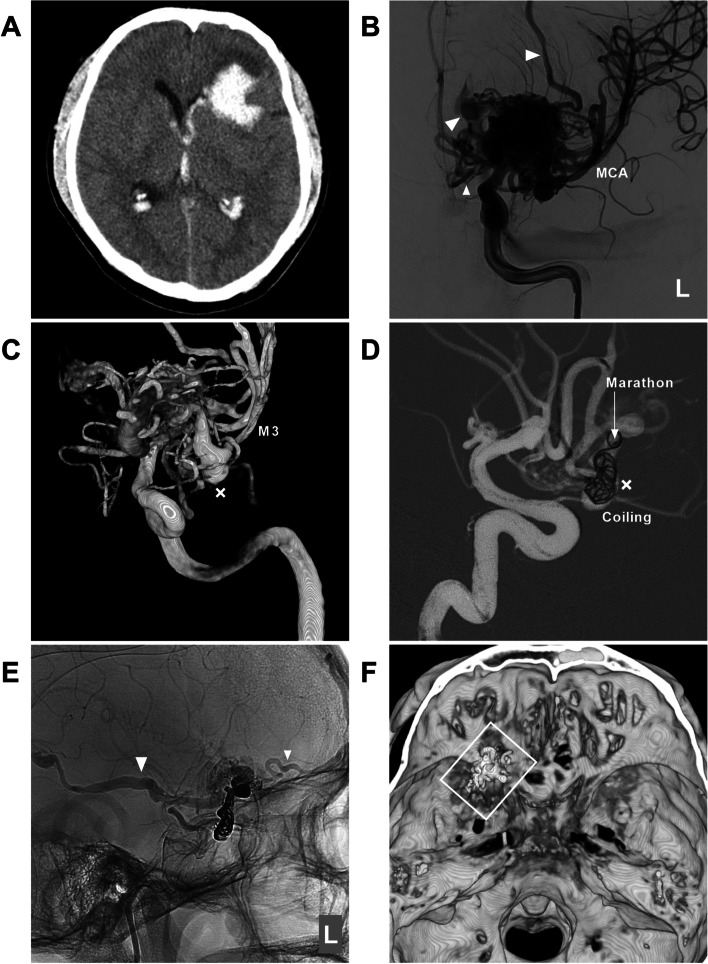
EVT assisted by the pressure cooker technique. A CT shows frontal hemorrhage above the Sylvian fissure. B-C: Two-dimensional angiogram (B) and three-dimensional reconstruction angiogram (C) of the left ICA show that the BAVM is supplied by the M3 segment multiple draining veins (arrowheads), and an aneurysm (fork in C) on the feeding artery. D Road-map navigation angiogram showing that the aneurysm (fork) has been coiled to create a pressure cooker effect; the tip of the Marathon microcatheter (arrow) is advanced beyond the coils. E Unsubtracted angiogram of the left ICA showing that the volume of the BAVM nidus is reduced, and the draining veins (arrowheads) are patent. E Reconstructive Xper CT showing the location of the Onyx (frame). Abbreviations: BAVM: brain arteriovenous malformation, CT: computed tomography, CTA: computed tomography angiography, EVT: endovascular treatment, ICA: internal carotid artery, L: left, MCA: middle cerebral artery, M3: third segment of the MCA
